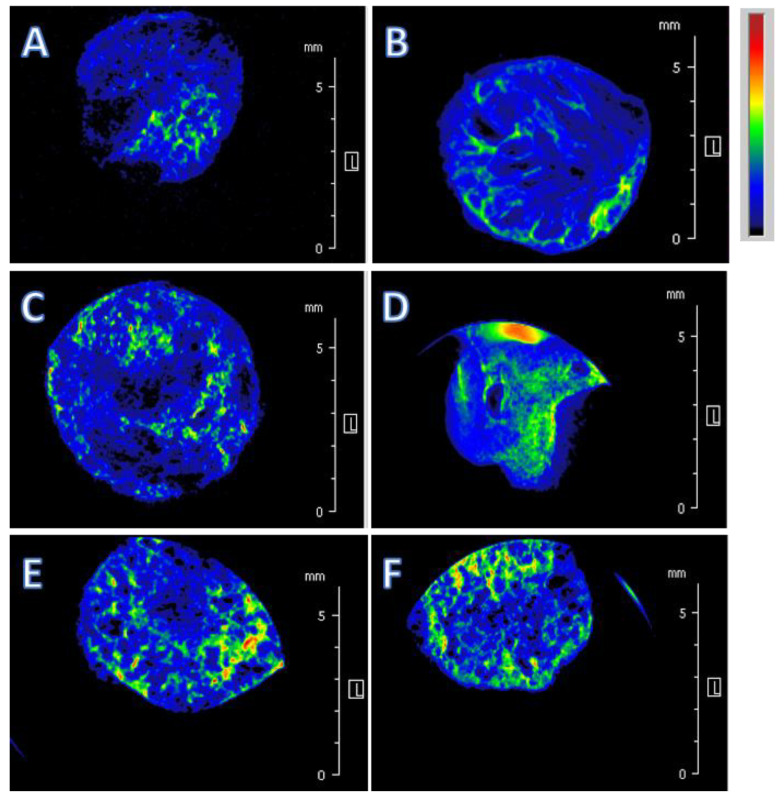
MRI images showing a representative central slice of each hydrogel after the addition of 150 μL of water necessary to saturate samples: (A) H-LMP, (B) H-HMP, (C) H-LMP-HS, (D) H-HMP-HS, (E) H-LMP-HULIS, (F) H-HMP-HULIS. The MRI images show the spatial distribution of water within the structure of samples, varying the color from blue to green and pale red as a function of increasing water content.
